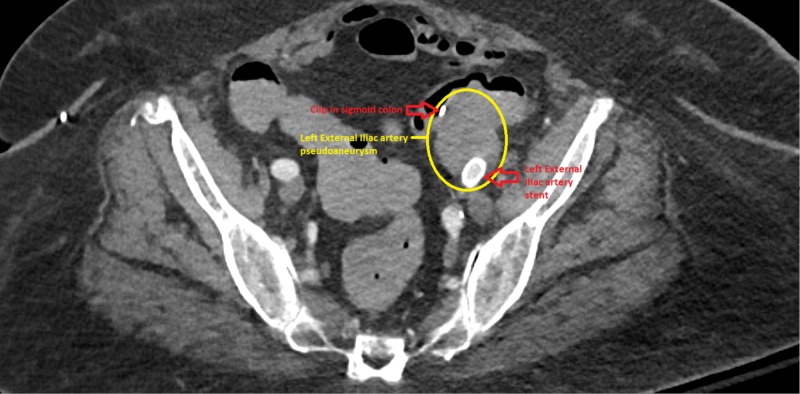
Post-operative computed tomography (CT) angiography of abdomen and pelvis shows patent left external iliac artery stent, with no endovascular leak
